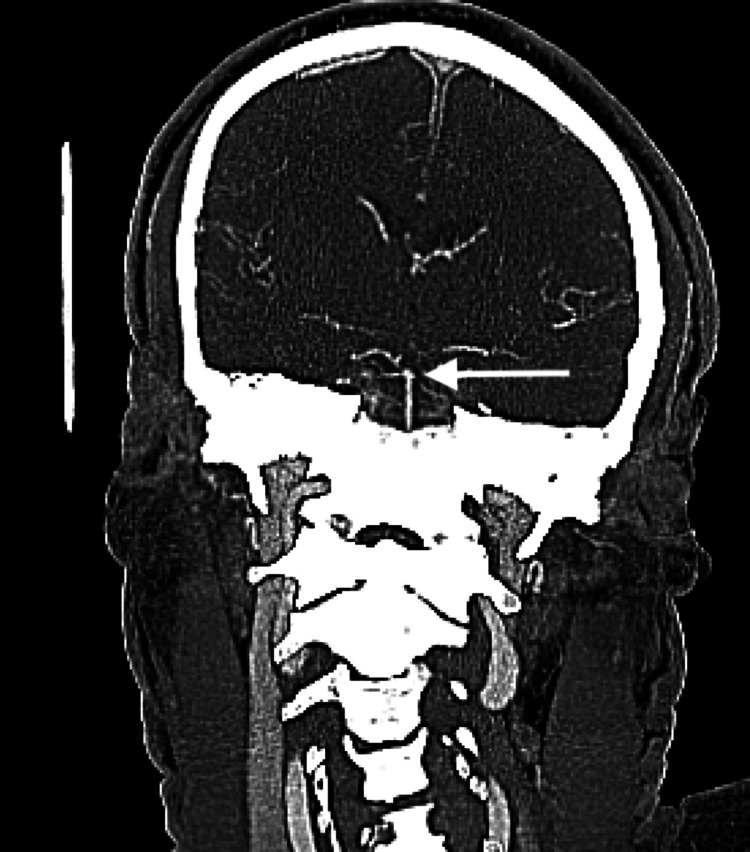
Computed tomography angiogram of the head, coronal view: top of the basilar artery appears patent.
